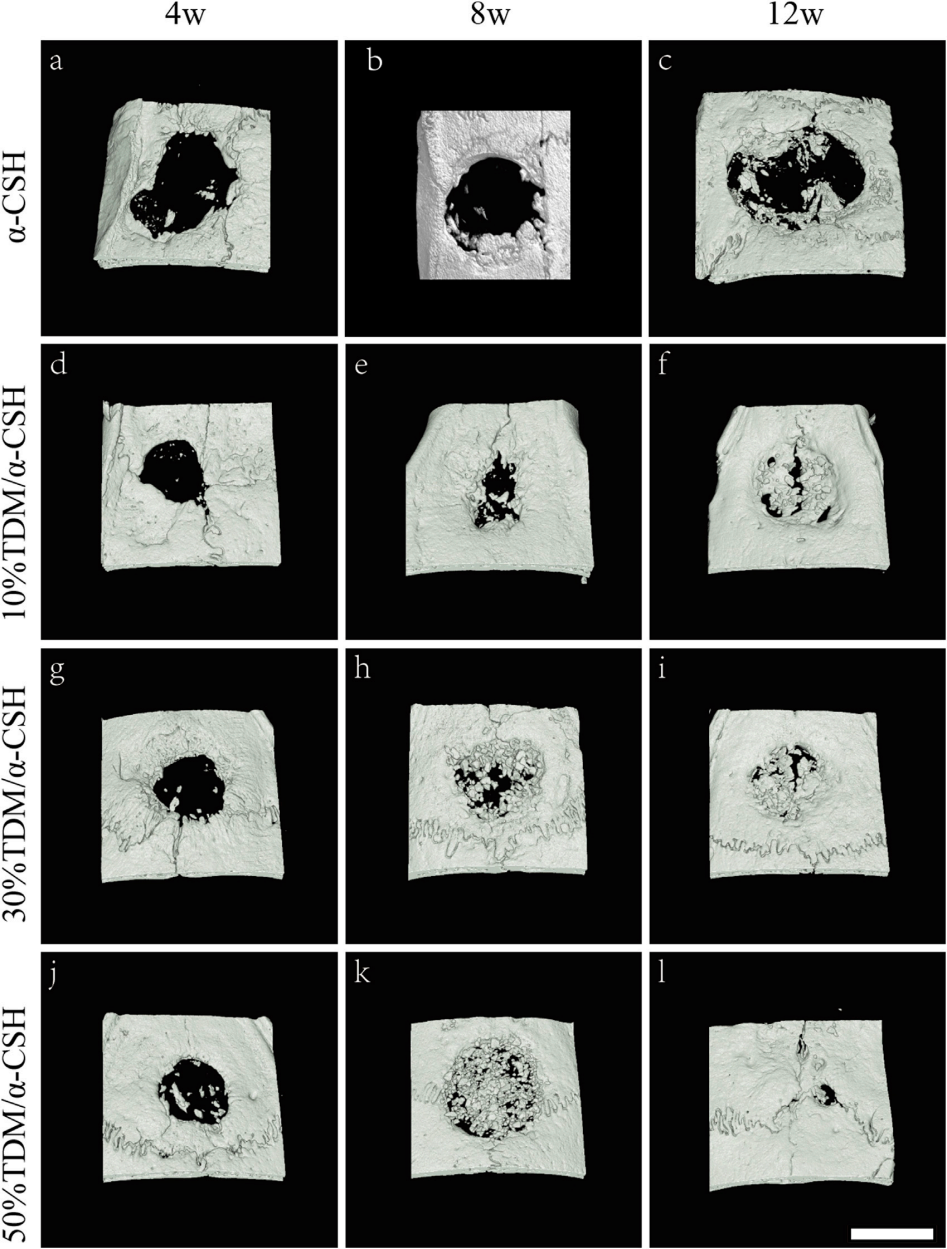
Three-dimensional reconstruction images of μ-CT scans showing critical-sized skull defects after the implantation of α-CSH (A–C), 10% TDM/α-CSH (D–F), 30% TDM/α-CSH (G–I), and 50% TDM/α-CSH (j–l) at 4 (A, D, G, J), 8 (B, E, H, K), and 12 (C, F, I, L) weeks post-surgery. Scale bar = 5 mm.
